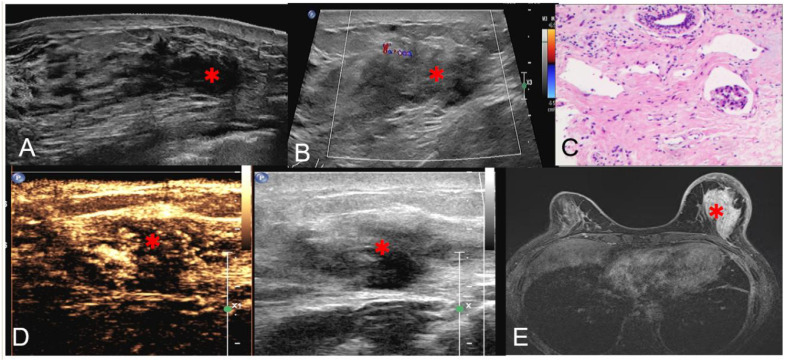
Pre-ablation imaging characteristics. (A) Panoramic imaging; (B) CDFI; (C) Pathological diagnosis; (D) Contrast-enhanced ultrasound(CEUS) demonstrating hypoenhanced lesion with extended enhancement; (E) MRI showing non-mass enhancement (*asterisk showed tumor area).
